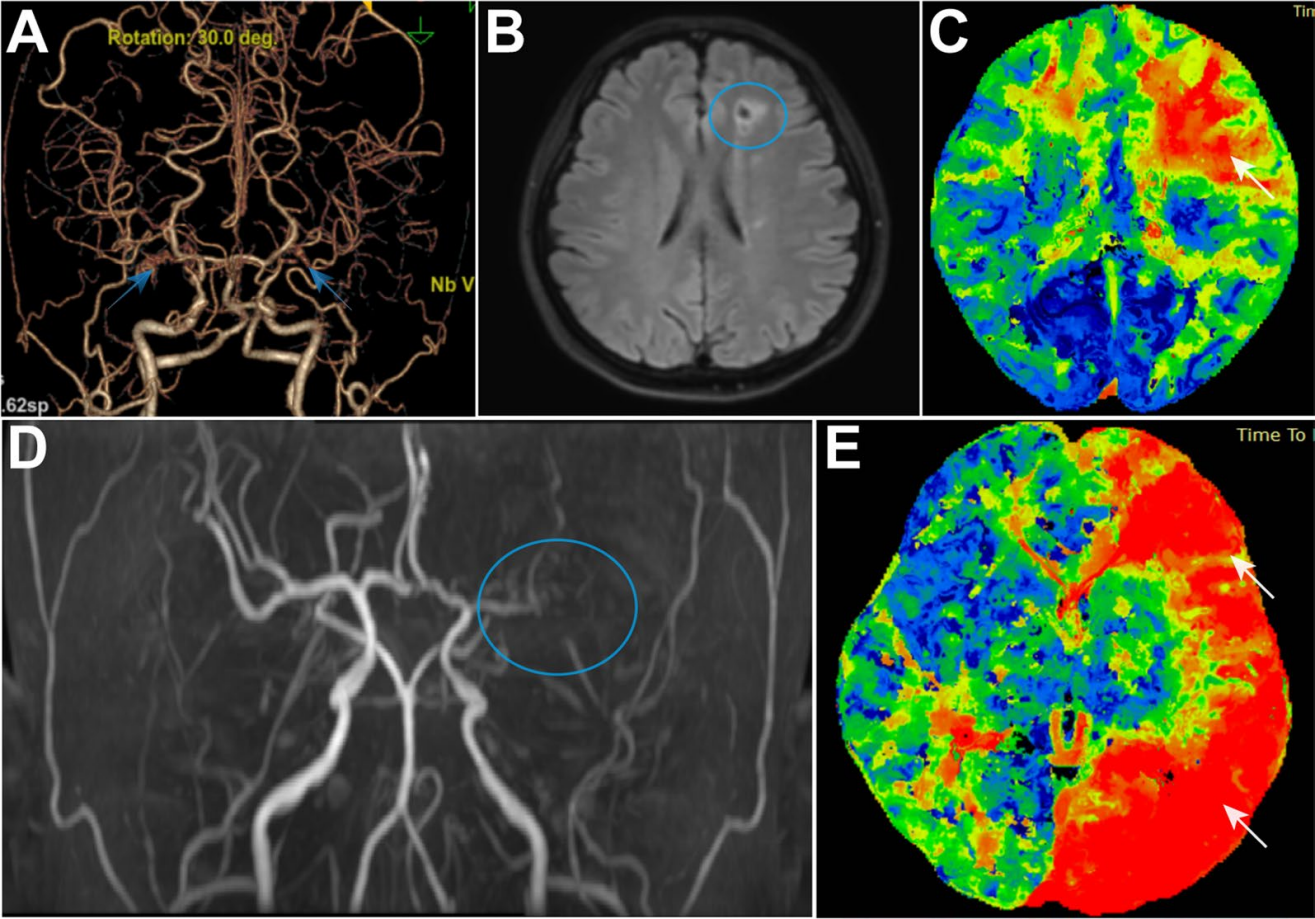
Typical image of bilateral and unilateral Moyamoya disease. A MRA images of cerebral vessels in a patient with bilateral Moya Moya disease. The blue arrow indicates the occluded middle cerebral artery. B MRI images of cerebral infarction in a patient with Moya Moya disease. The blue circle indicates the area of cerebral infarction. C Cerebral perfusion images of a patient with Moya Moya disease. The red area represents the low perfusion region, which coincides with the location of cerebral infarction. D CTA images of cerebral vessels in a patient with unilateral Moya Moya disease. The blue circle indicates the occluded middle cerebral artery. E Cerebral perfusion images of a patient with unilateral Moya Moya disease. The red area represents the low perfusion region, which is consistent with the side of the occluded middle cerebral artery
